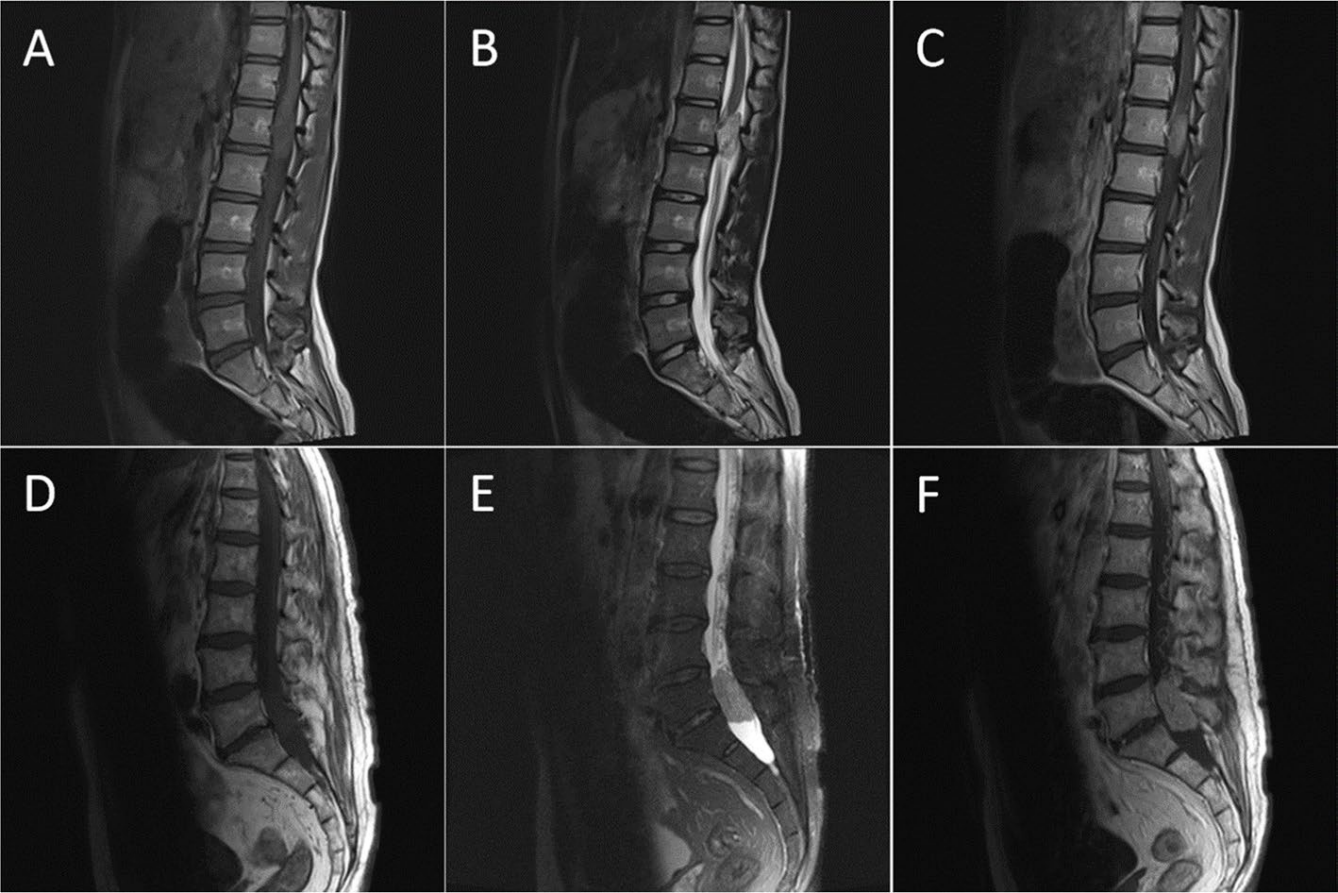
Enhanced MR images of ependymoma and schwannoma. Images A-C belong to a 13-year-old female patient who was admitted to the hospital due to left lower limb pain. Postoperative pathology indicated a spinal ependymoma, WHO Grade II. Images D-F belong to a 55-year-old male patient who was admitted to the hospital due to lower back pain and left lower limb pain. Postoperative pathology indicated a schwannoma. A & D T1-weighted image (T1-WI). B & E T2-weighted image (T2-WI). C & F Contrast-enhanced T1-weighted image (CE-T1)
